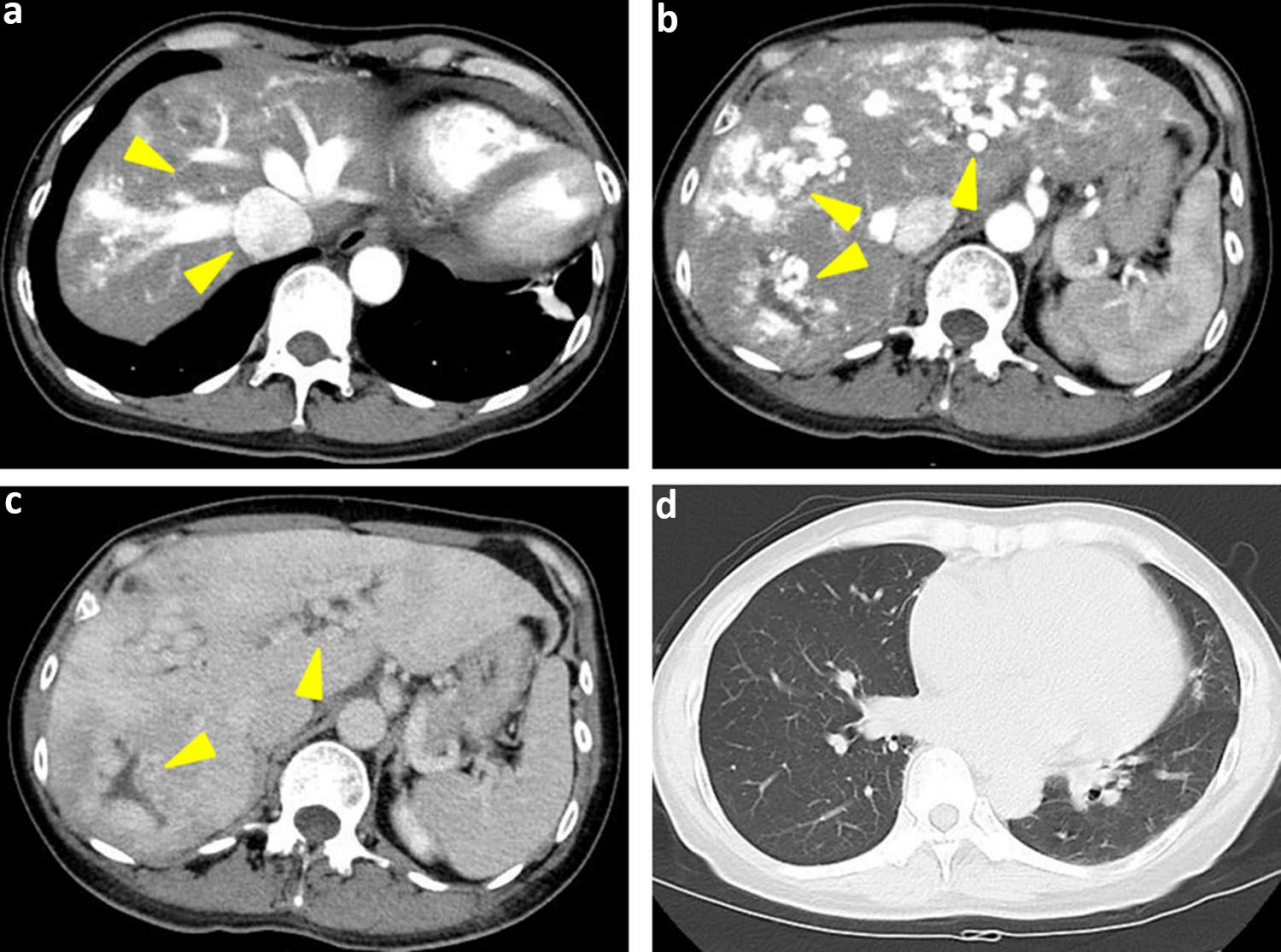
Contrast-enhanced computed tomography (June 20XX + 3) a Marked intrahepatic dilation of the hepatic vein (HV) and inferior vena cava (IVC) is observed during the arterial phase. b Dilated and tortuous arteries are observed in both lobes of the liver. c In the equilibrium phase, dilated bile ducts can be seen in both lobes of the liver. d No arteriovenous malformations were found in the lungs.
